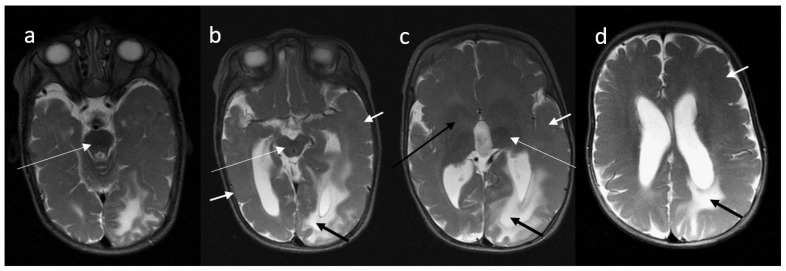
A 9-month-old boy, MR brain examination, (a–d)—axial T2-weighted images. T2-weighted images in axial plane demonstrate asymmetry of the cerebral hemispheres with the left one slightly larger than right. Diffuse loss of sulcation, broad gyri in association with diffuse polymicrogyria/pachygyria and indistinct gray–white matter differentiation are seen in both cerebral hemispheres (short white arrows on (b–d)). Lack of white matter myelination: weak myelination is visible only in posterior limbs of the internal capsules (long white arrow on (c)) and brainstem (long white arrows on (a,b)) and cerebellar peduncles (not shown). Basal ganglia show abnormal appearance bilaterally (long black arrow on (c)). The white matter of the left occipital lobe and posterior part of left parietal and frontal lobes is abnormally hyperintense on T2-WI (short black arrows on (b–d)). Brain stem (long white arrows on (a,b)) and splenium of the corpus callosum are hypoplastic. The lateral ventricles and third ventricle are enlarged.
